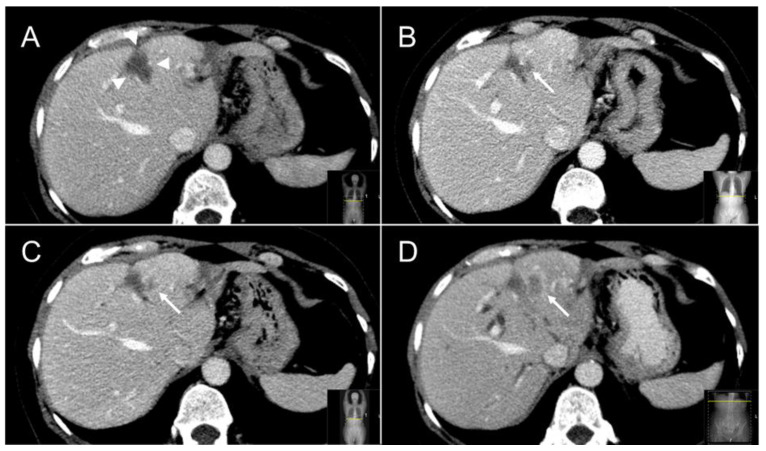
Follow-up cross-sectional ceCT images of a CRLM treated with thermal ablation. At six months a typical sharply demarcated hypoattenuating scar lesion (white arrowheads) is visible (A). At 12 months a characteristic “incomplete ring sign” (white arrow) at the left margin of the ablation zone can be appreciated (B), which, especially when combined with 18F-FDG avidity, is highly specific for local tumor progression following thermal ablation. Follow-up ceCT at 15 and 18 months (C,D) further confirmed local tumor progression.
